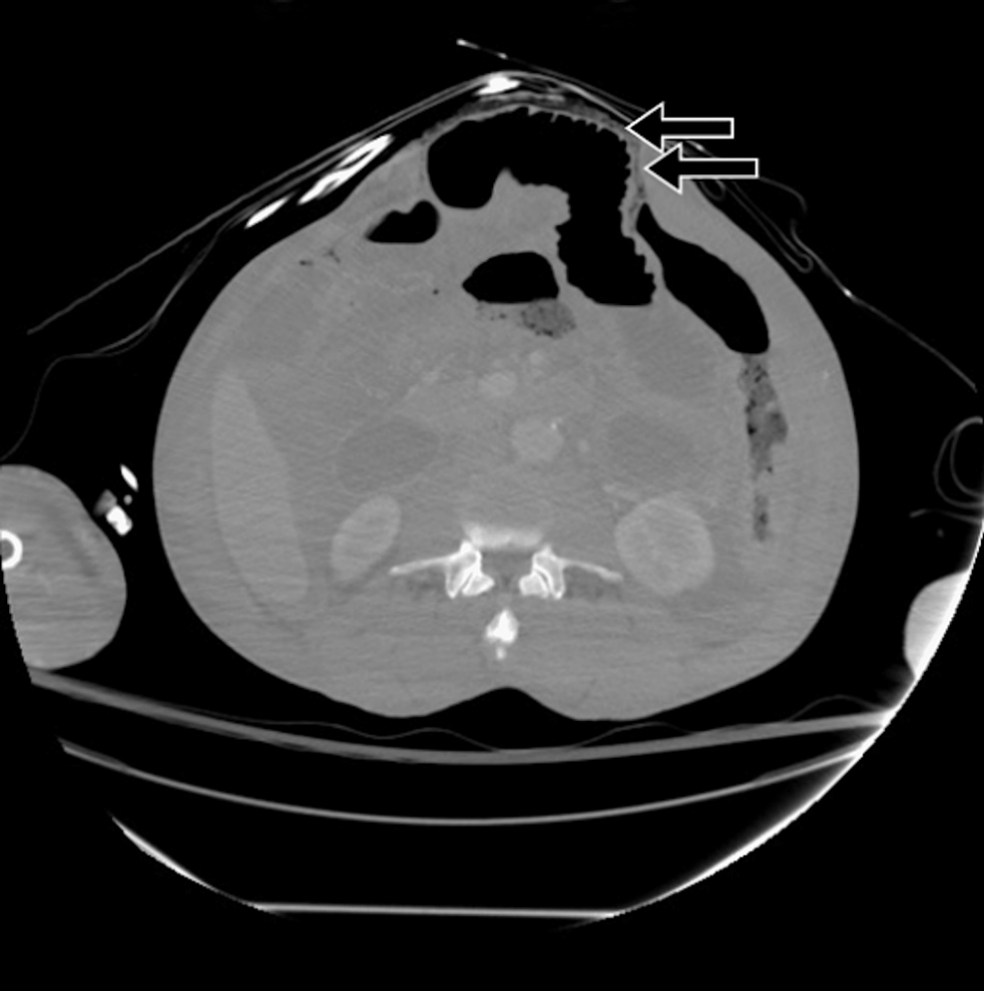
Abdomen/pelvis CT demonstrating pneumatosis of the small bowel, suggestive of ischemia (arrows).
